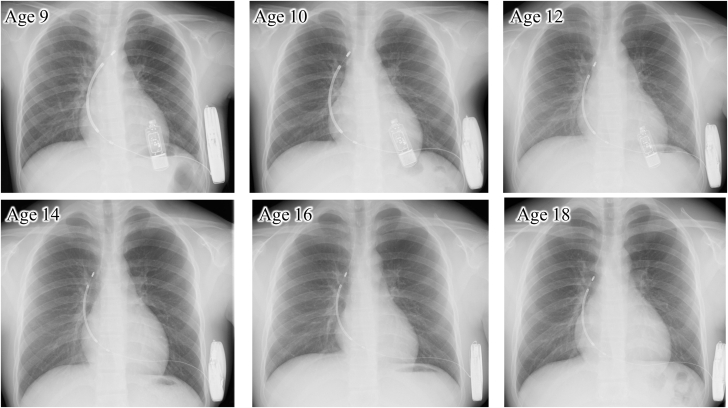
Serial Chest Radiographs During Growth Chest radiographs obtained at ages 9, 10, 12, 14, 16, and 18 years demonstrate stable positioning of the subcutaneous implantable cardioverter-defibrillator lead over time. The C-curve lead placement with intentional slack successfully accommodated a height increase of more than 35 cm during growth.
